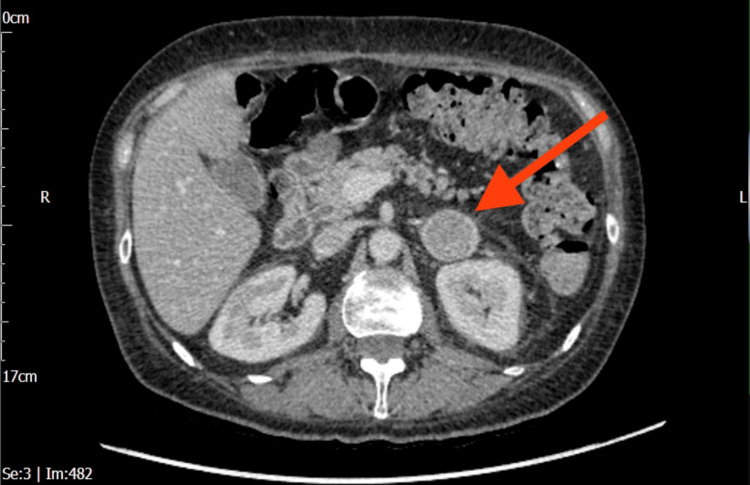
Triple-phase CT of the abdomen demonstrating a left adrenal mass (red arrow). Note the preserved volume of the contralateral adrenal gland CT: computed tomography
